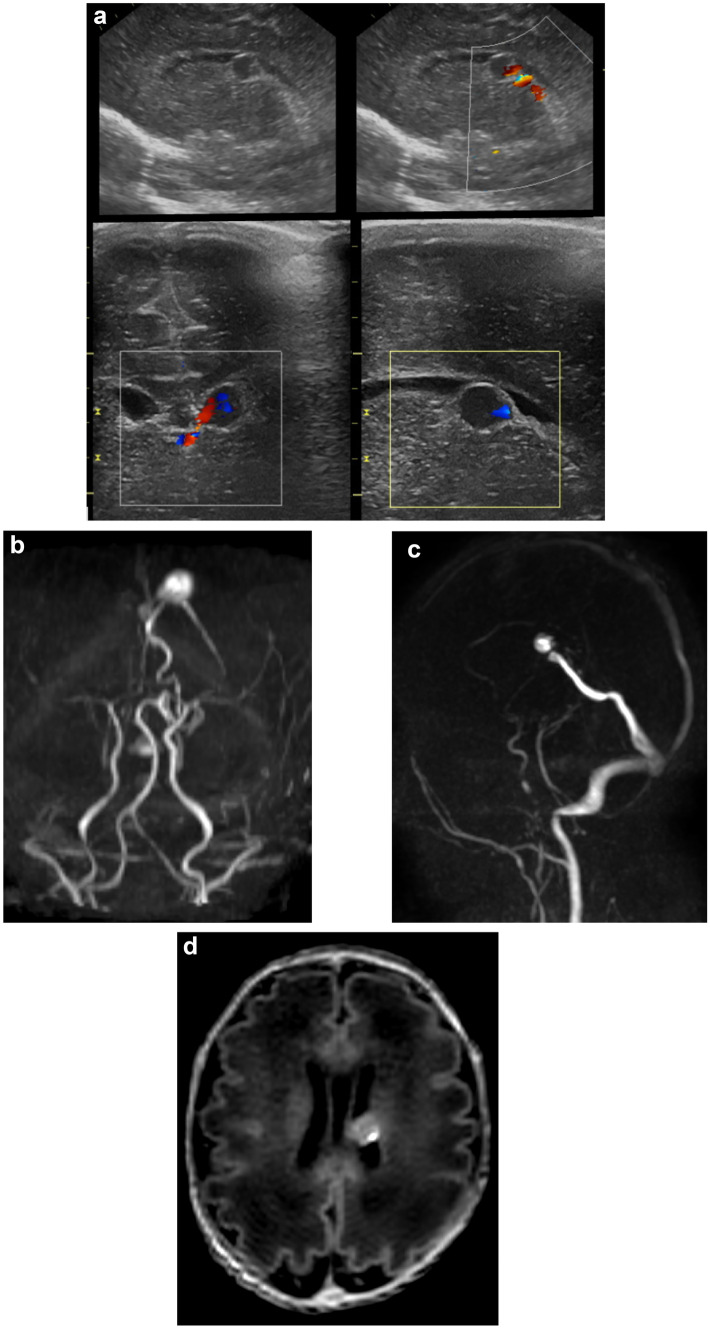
a Cerebral ultrasound demonstrating a “cystic” lesion in the left germinal matrix with flow on color Doppler mode. b A ToF MR angiography in the arterial phase shows arterial flow in the pouch and the presence of a fistula with two feeders from the posterior cerebral arteries. c A single deep venous drainage into the vein of Galen is noted. d A T1-weighted MR image demonstrates hyperintensity of the posterior wall of the pouch due to partial thrombosis.
